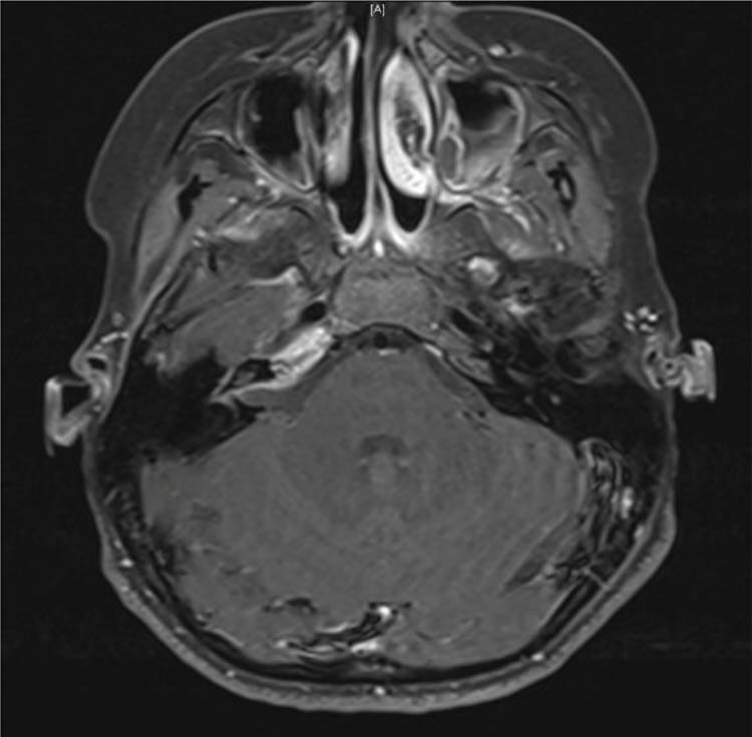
Axial contrast-enhanced T1-weighted image taken 3 months after admission shows enhancement within the right petrous apex and anterior aspect of the internal auditory canal.
